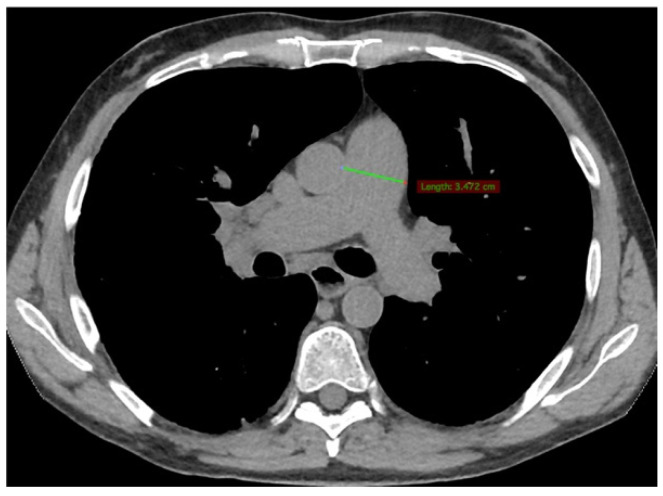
Dilated pulmonary artery trunk at repeated HRCT.
